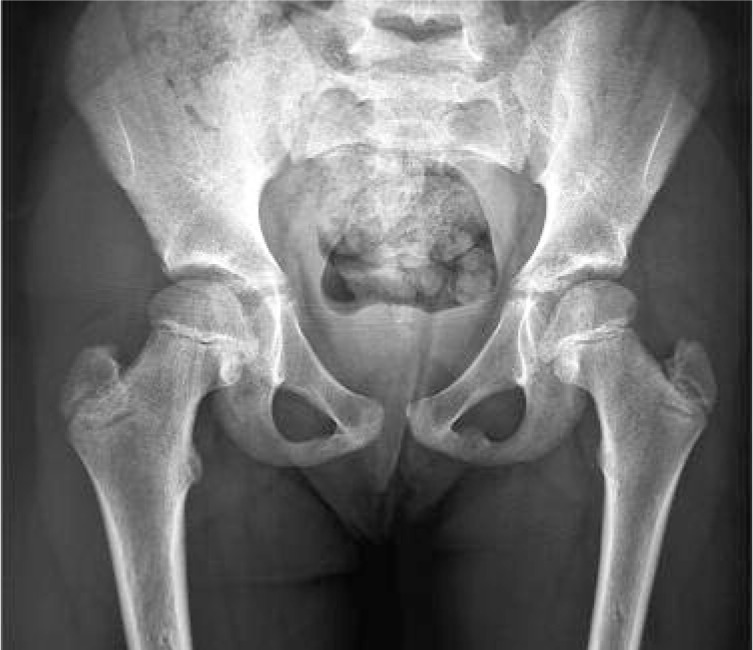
Post reduction radiograph showing good reduction of the hip.
